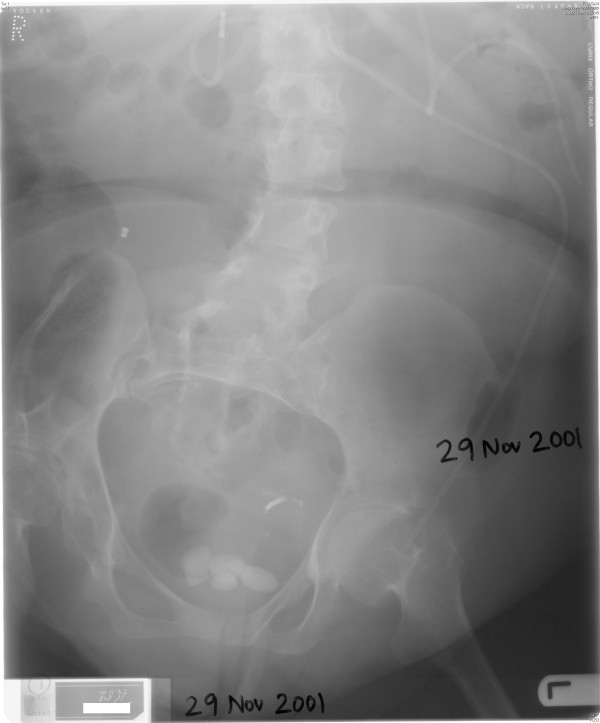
X-ray of abdomen, taken on 29 November 2001, showed several vesical calculi.
